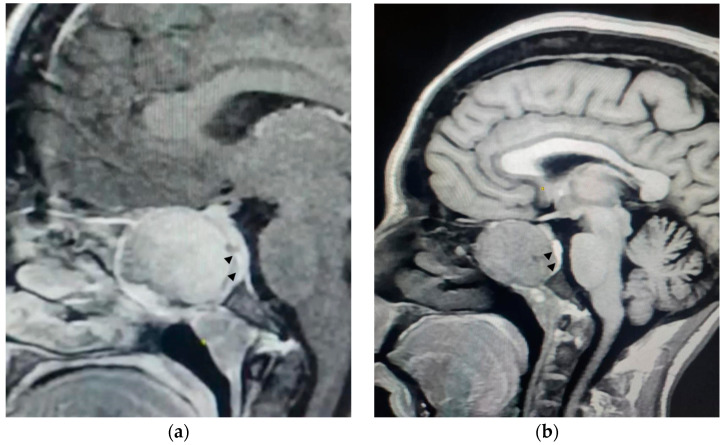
(a) A T1-weighted sagittal MRI with a gadolinium injection confirms the tissue nature of the mass and its expansion against the bony cortex (arrowheads). (b) A T1-weighted sagittal MRI without a gadolinium injection shows a lesion centered on the sphenoid sinus, expanding the bony cortex of the sinus, displacing the flattened pituitary gland posteriorly, filling the opto-chiasmatic cisterns superiorly, and making intimate contact with the optic nerve.
